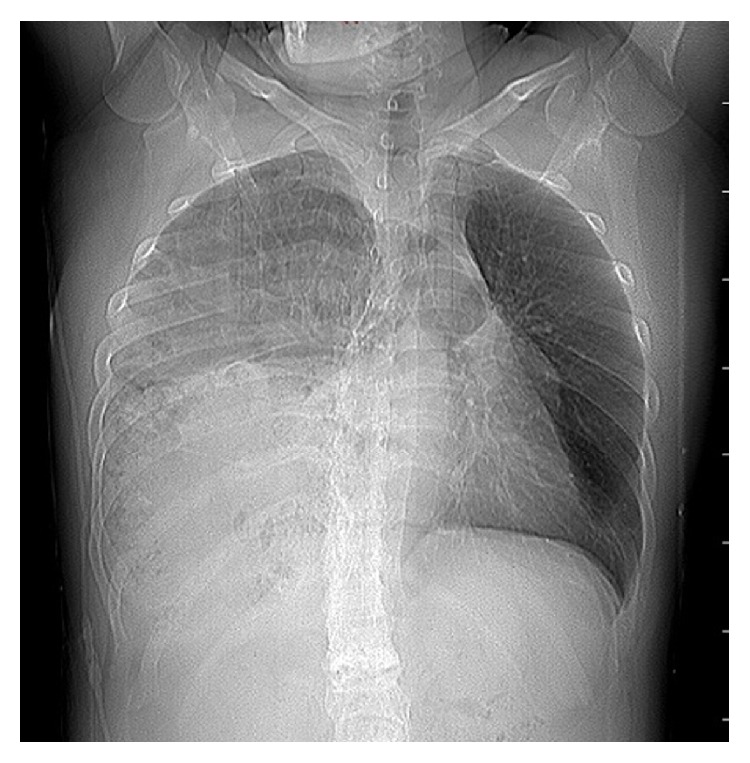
Chest X-ray. Right lung was pushed upwards and right hemithorax was filled with intestines.
